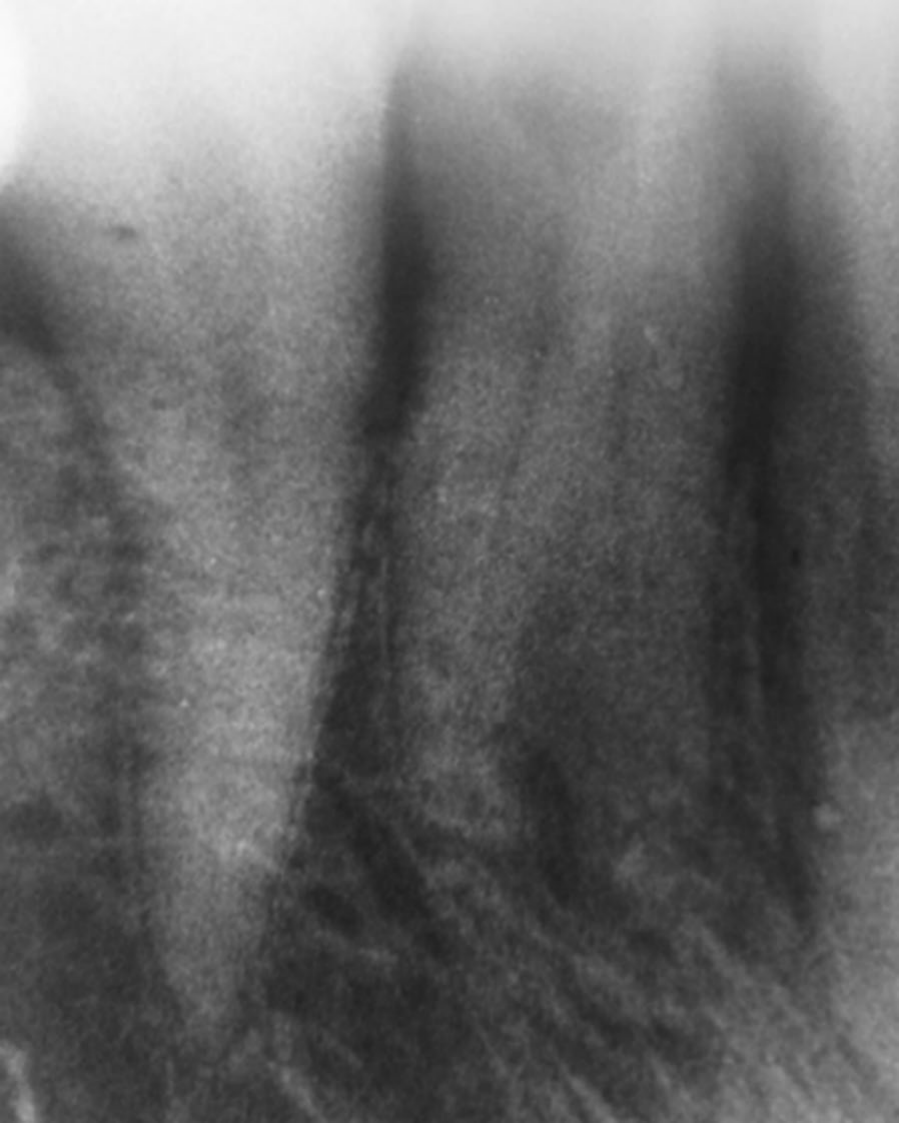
Diagnostic radiograph showing two roots
